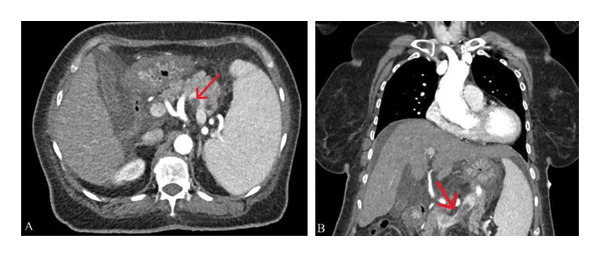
Axial (A) and coronal (B) contrast‐enhanced chest CT scans showing filling defects within the splenic vein.
